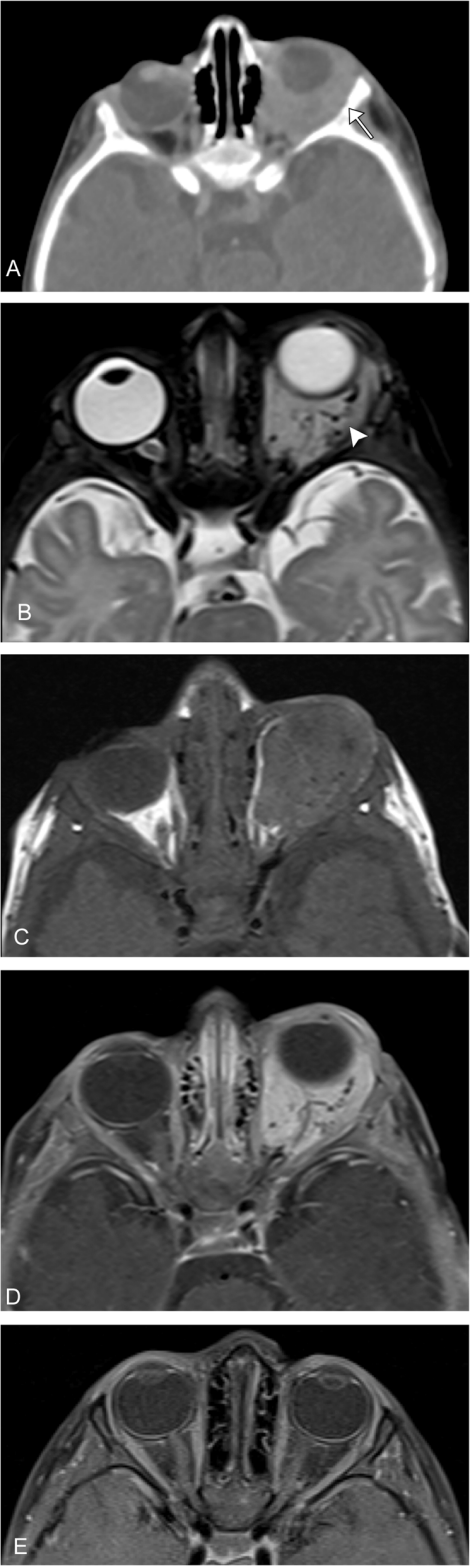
Infantile hemangioma. A 10-week-old full-term healthy infant presents with 1 week history of gradual swelling of the left eye. a Axial CT with contrast demonstrates an enhancing mass infiltrating the left intraorbital soft tissues, with resultant proptosis and smooth scalloping of the lateral orbital wall (arrow). b Axial T2 image demonstrates a mildly hyperintense mass (note: not as bright as cerebral spinal fluid) with prominent serpiginous vascular flow voids (arrowhead). c Axial T1 pre- and (d) post-contrast T1 fat suppressed images demonstrate avid enhancement of the lesion. e The patient was treated with propranol and post-contrast T1 fat suppressed imaging demonstrates resolution of the lesion
